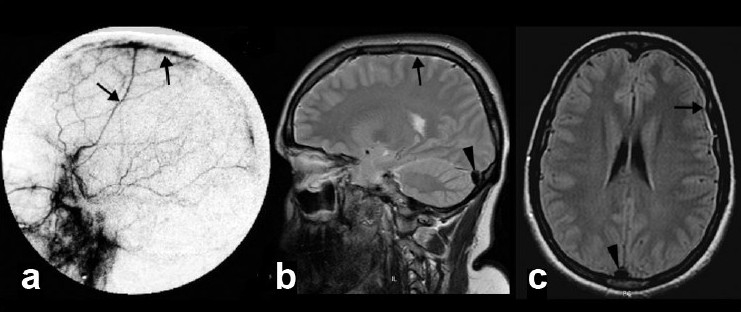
Lateral view of digital subtraction angiography of left carotid angiogram (a) shows increased vascularity and early filling of the pachymeninges covering the sagittal sinus through meningeal arteries (arrow). This appearance is due to meningeal vasodilatation. Sagittal T2 weighted (b) and axial FLAIR (c) images show pachymeningeal T2 hyperintensity (black arrow) and venous distension sign (black arrowhead)
